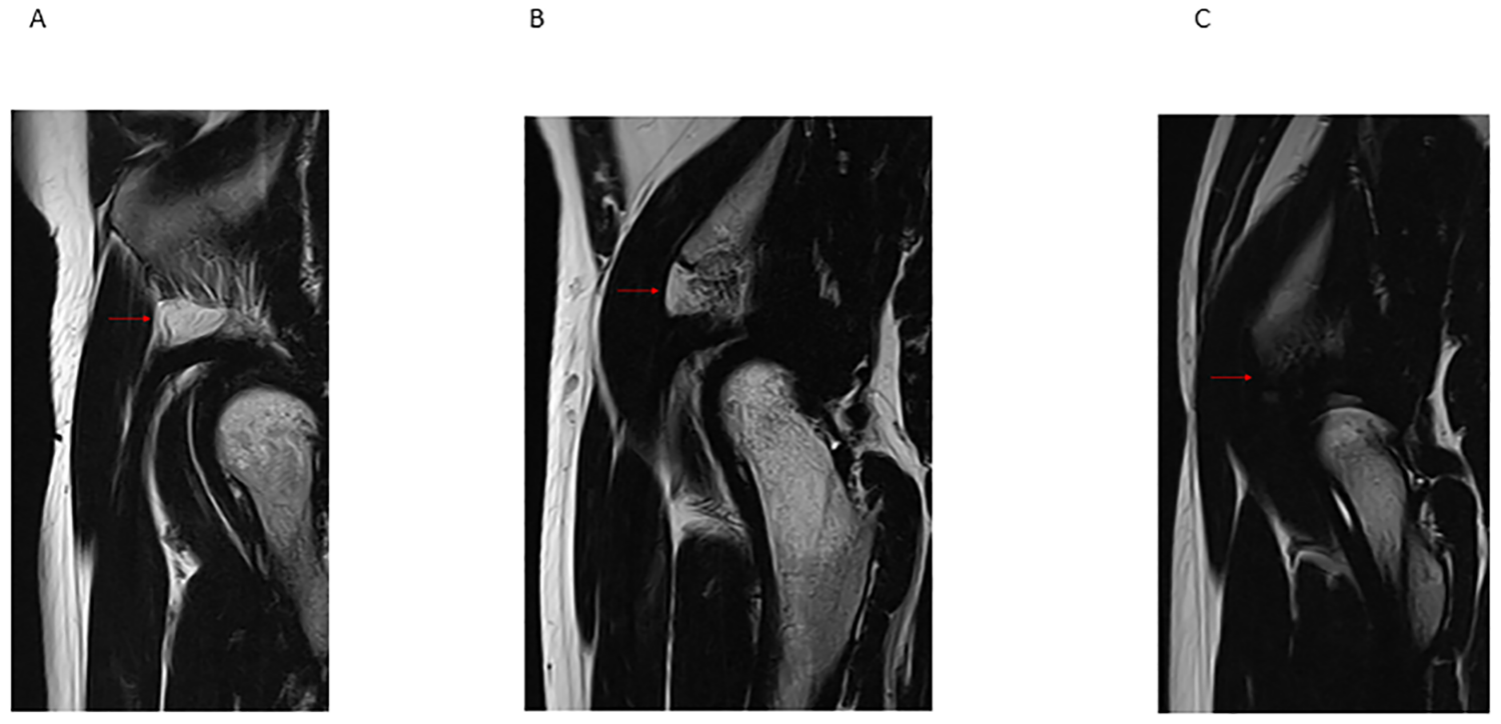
T2-weighted sagittal image of hip joint. Normal fat pad (A, red arrow), fat pad with fibrosis (B, red arrow), fibrous scar formation (C, red arrow).
